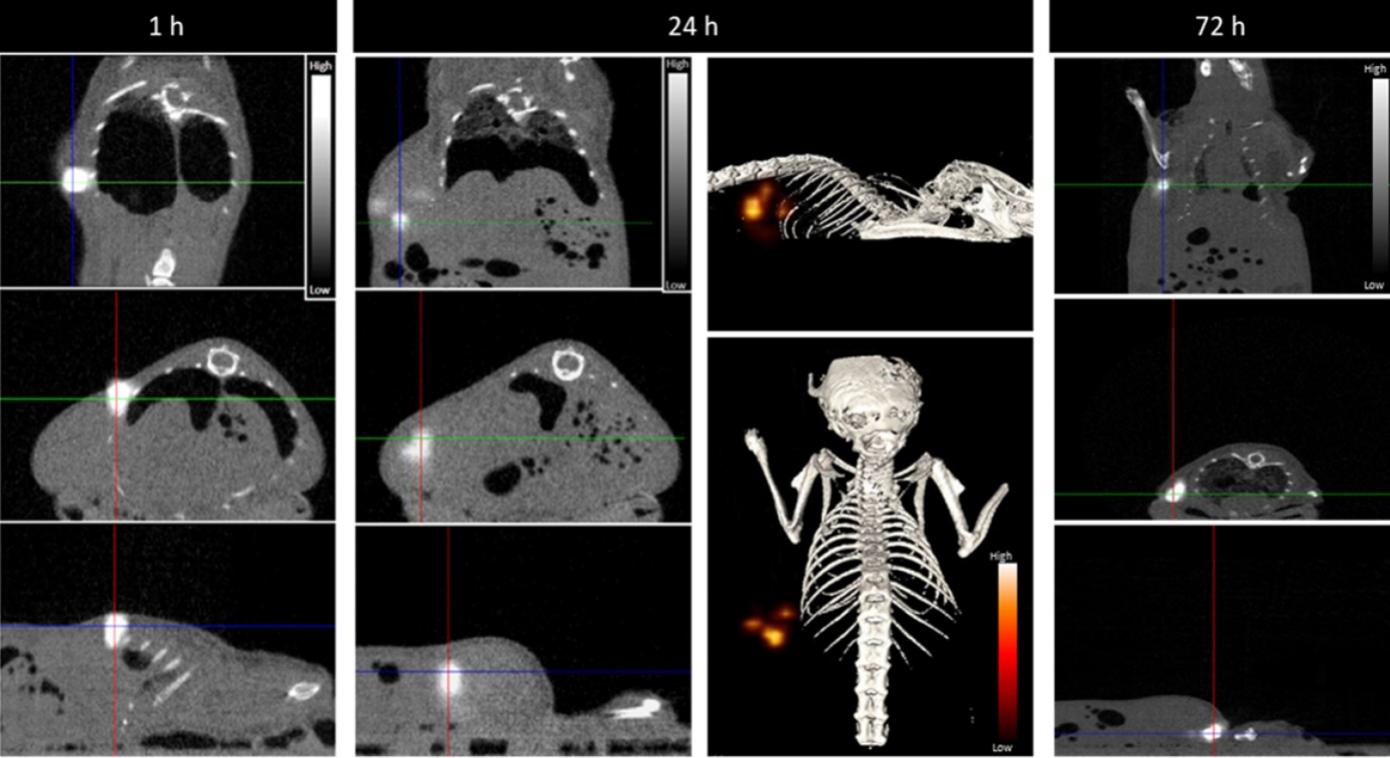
Serial SPECT/CT imaging at 1, 24, and 72 h after intratumoral injection of ^67^Ga-AuNP-BBN-Pt1. The gray scale images represent the registered SPECT-CT slices at indicated times, respectively, the coronal (top), transaxial (center) and sagittal (bottom) planes. The color scale images represent a 3D view of the ^67^Ga activity, reflecting the tissue distribution studies. Intensity bars represent a range of intensities (arbitrary units) for all panels, which have been normalized to same intensity scale.
